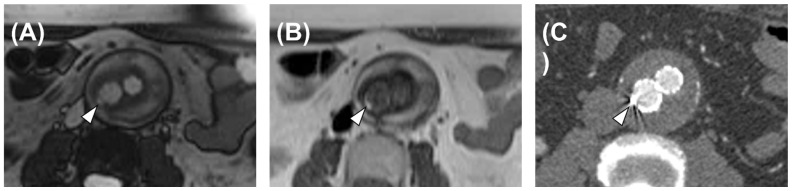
An example of a false-positive case, a 78-year-old male patient. (A) True-FISP sequence: hyperintensity can be observed next to the wall of the endograft (arrowhead). (B) HASTE sequence: hyperintensity can be observed next to the wall of the endograft (arrowhead). (C) CTA: arterial phase acquisition. A metallic artifact can be observed in correspondence with RM hyperintensities (arrowhead), possibly causing the misinterpretation of the RM images.
